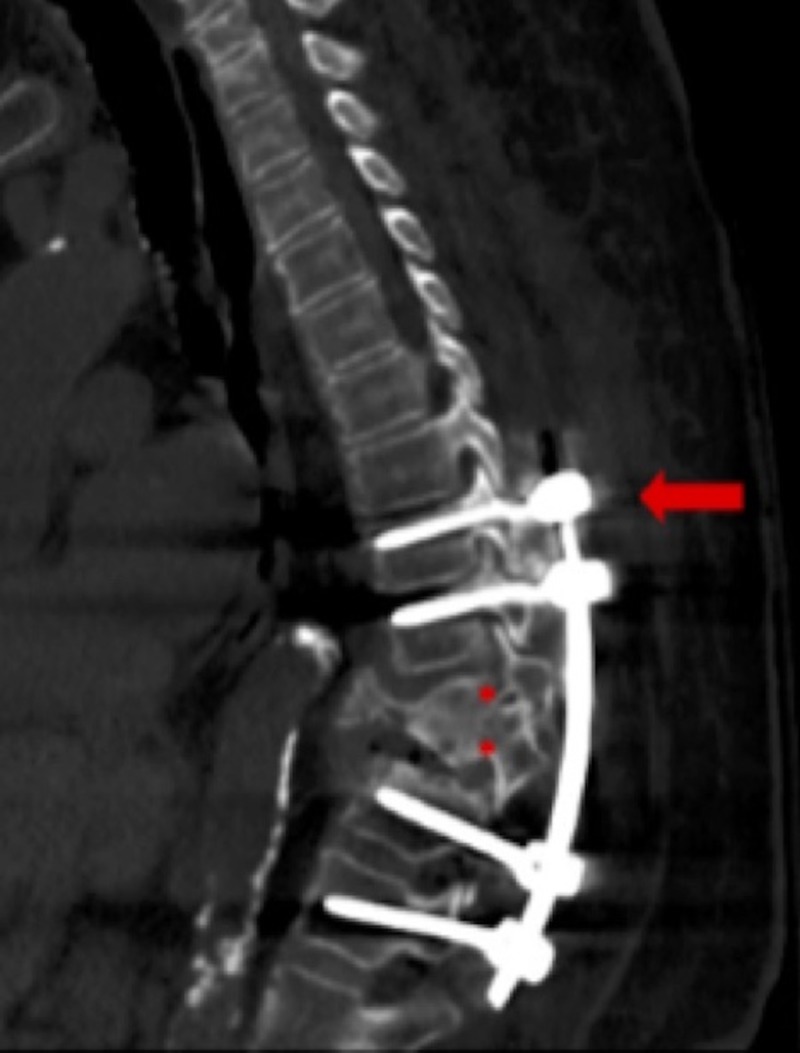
Computed tomography (CT) of the chest two months postoperatively Sagittal view demonstrating proper placement of pedicle screws at T9 (arrow), T10, T12, and L1. The duplicated T11 pedicle is also seen on this sagittal projection (asterisks).
